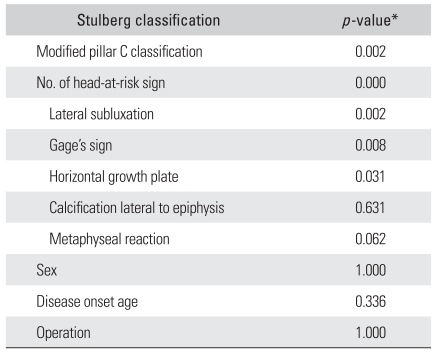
Relationship between Stulberg Classification and the Demographic, Radiographic and Clinical Data.
